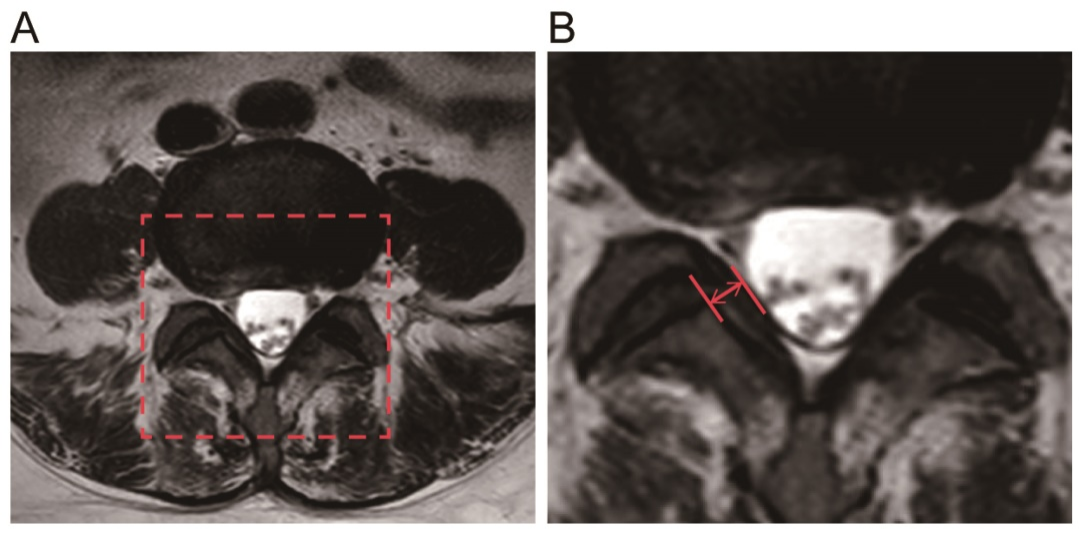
Measurement of the LF thickness by MRI. A The red frame was selected as the target analysis area. B The red arrow indicates the thickness of the ligamentum flavum at the facet joint level.
